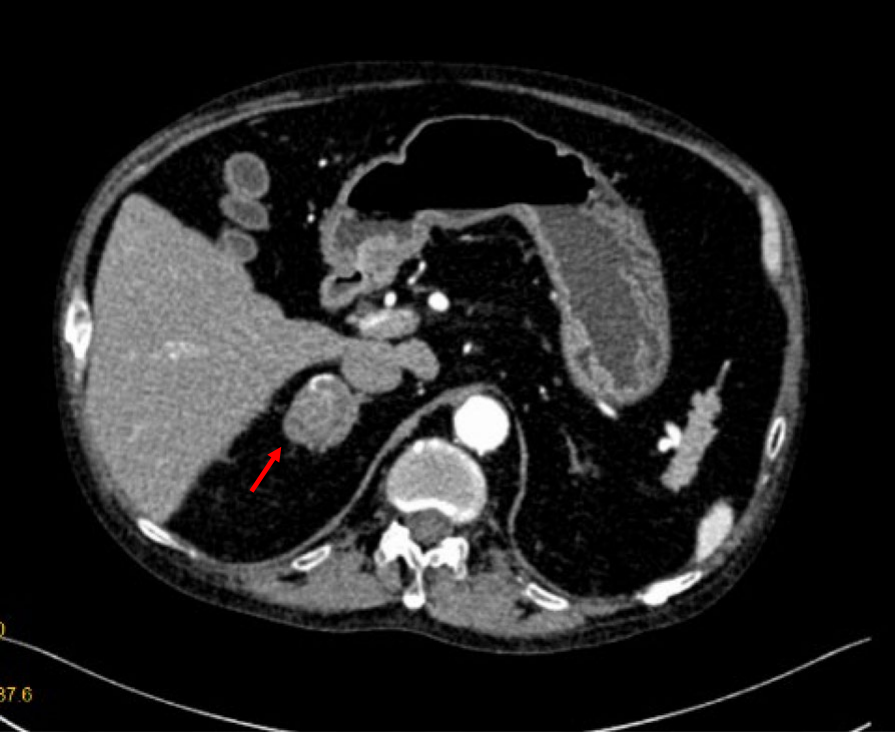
Computed tomography scan of the abdomen showed a 3 × 3 cm right adrenal mass as indicated by the red arrow
